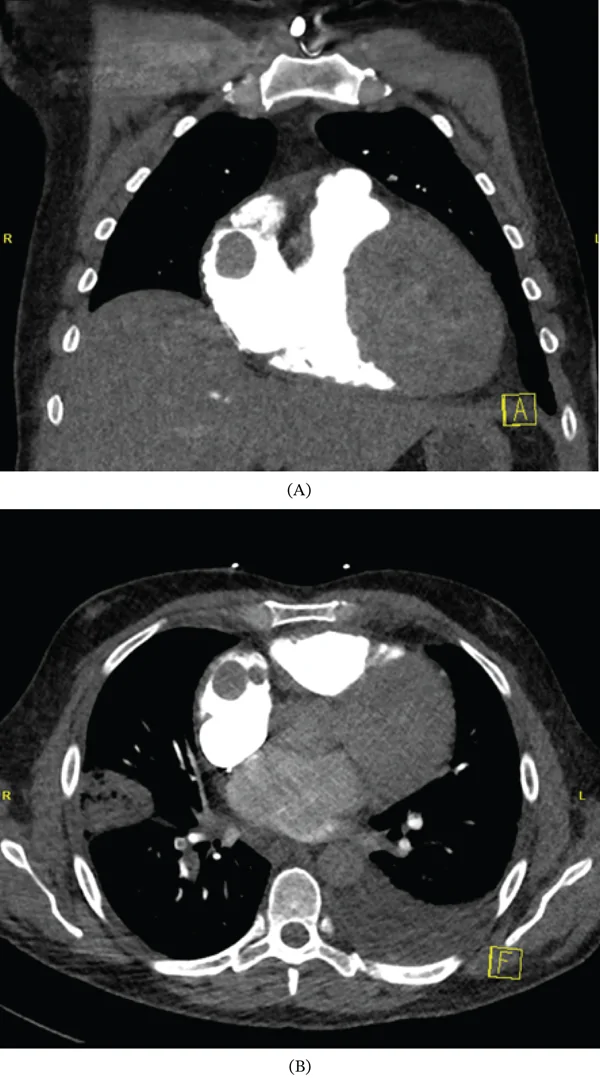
CT‐pulmonary angiogram on day of admission. (A) Coronal view. (B) Axial view.
